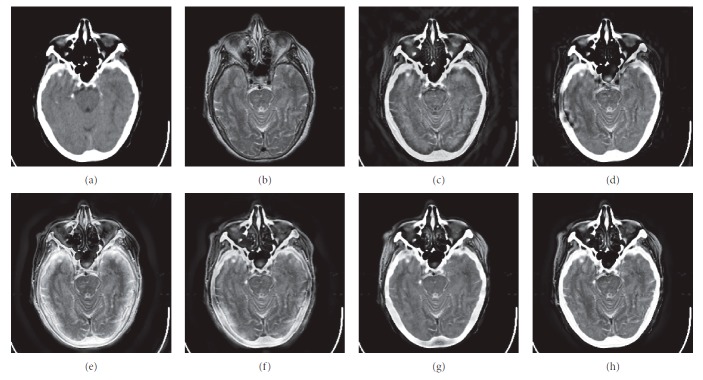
CT/MR-T2 medical image fusion results. (a) CT original image. (b) MR-T2 original image. (c) Method 1. (d) Method 2. (e) Method 3. (f) Method 4. (g) Method 5. (h) FFST-SR-PCNN.
